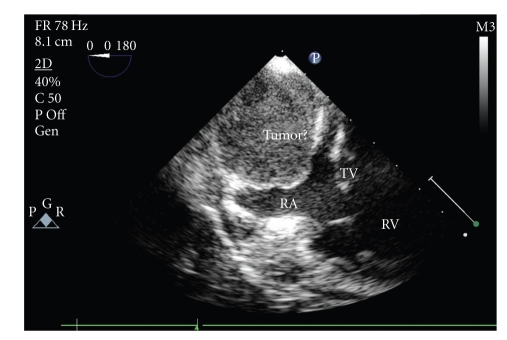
Transesophageal echocardiogram showing the homogenous echogenic mass (tumor) attached to the posterior wall of the right atrium (RA) above the tricuspid valve (TV), RV: right ventricle.
